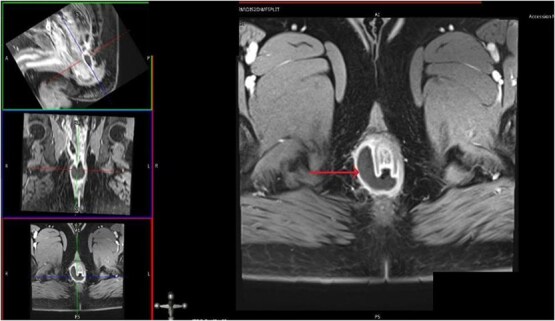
MRI of the anus revealing right inferior abscess (arrow).
